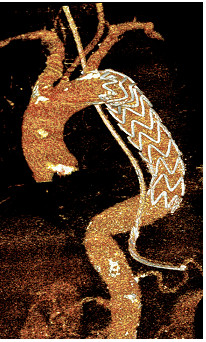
CT scan reconstruction shows regular closure of the entry site by the stent graft.
